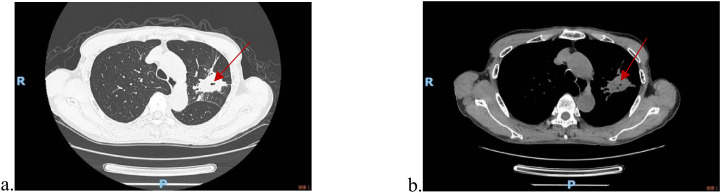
Patient CT at initial diagnosis (a) and contrast-enhanced CT (b).
